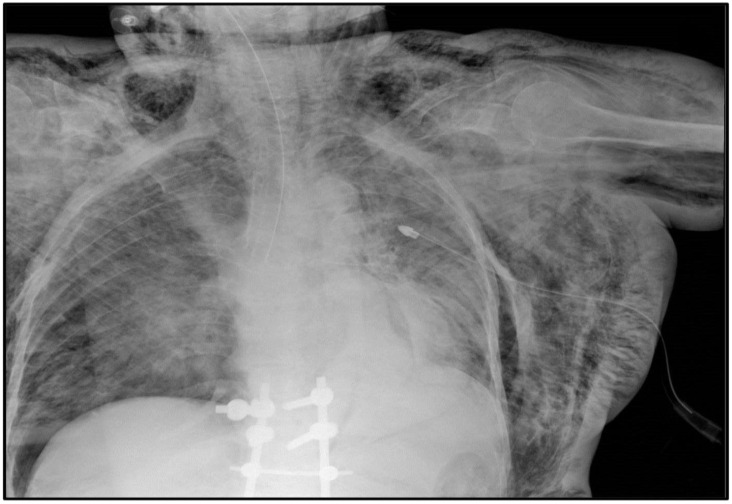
Chest X-ray of case #2 showing bilateral pneumothorax, pneumomediastinum and massive subcutaneous thoraco-abdominal emphysema extended to the upper limbs and neck; a left pleural drainage, the orotracheal tube and spinal surgery outcomes are well observable too.
